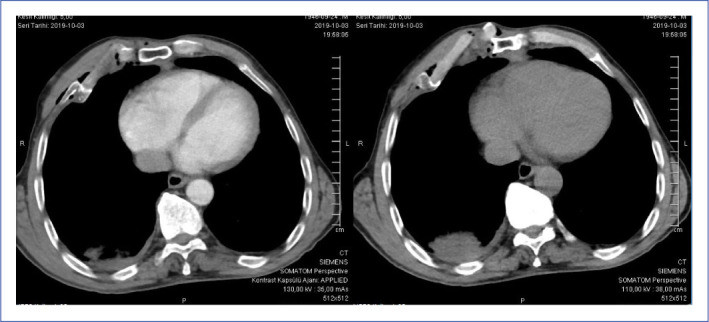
Thoracic CT of a flail chest case with rib fracture.
